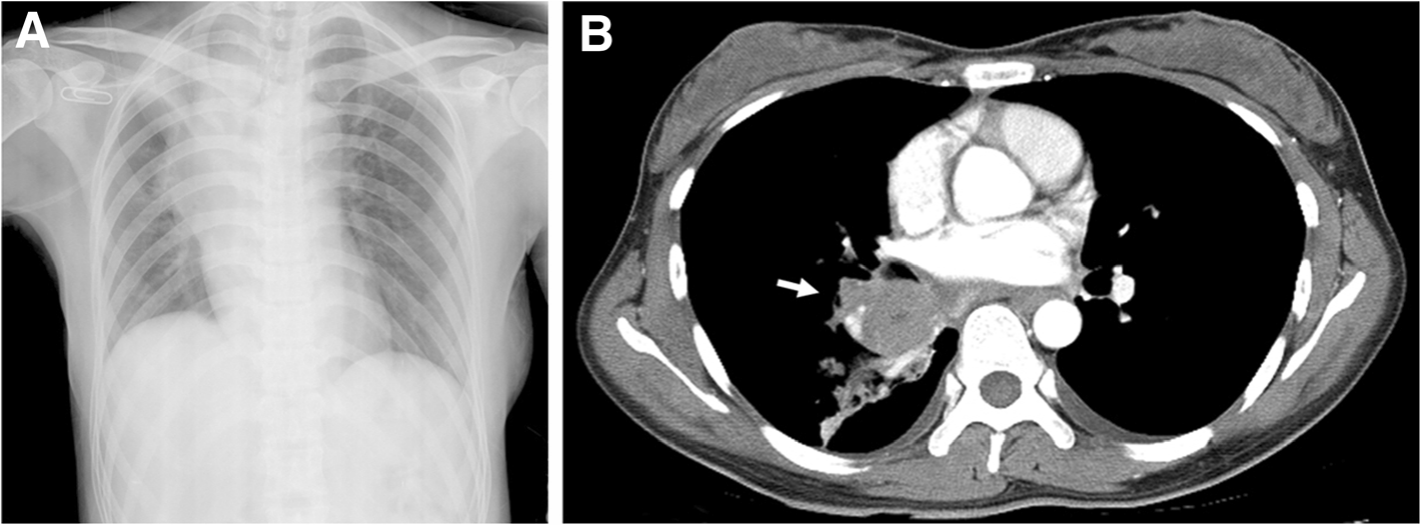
Chest radiograph and lung CT scan. A: Chest radiograph showing widened mediastinum in the upper-middle lobe and upper lobe atelectasis in the right lung, indicating a space-occupying lesion. B: Lung CT scan showing a right hilar mass measuring about 5.0 × 4.8 cm (arrow).
